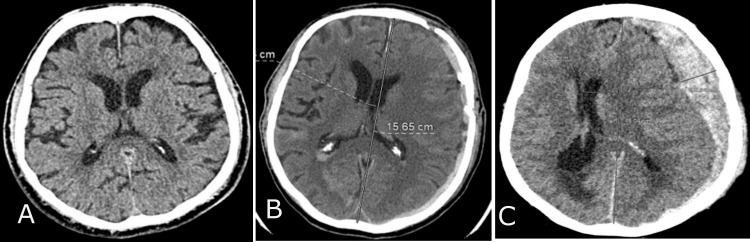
Midline shift CT findings Midline shift normal findings (A); midline shift ≤5 mm (B); midline shift >5 mm (C).
